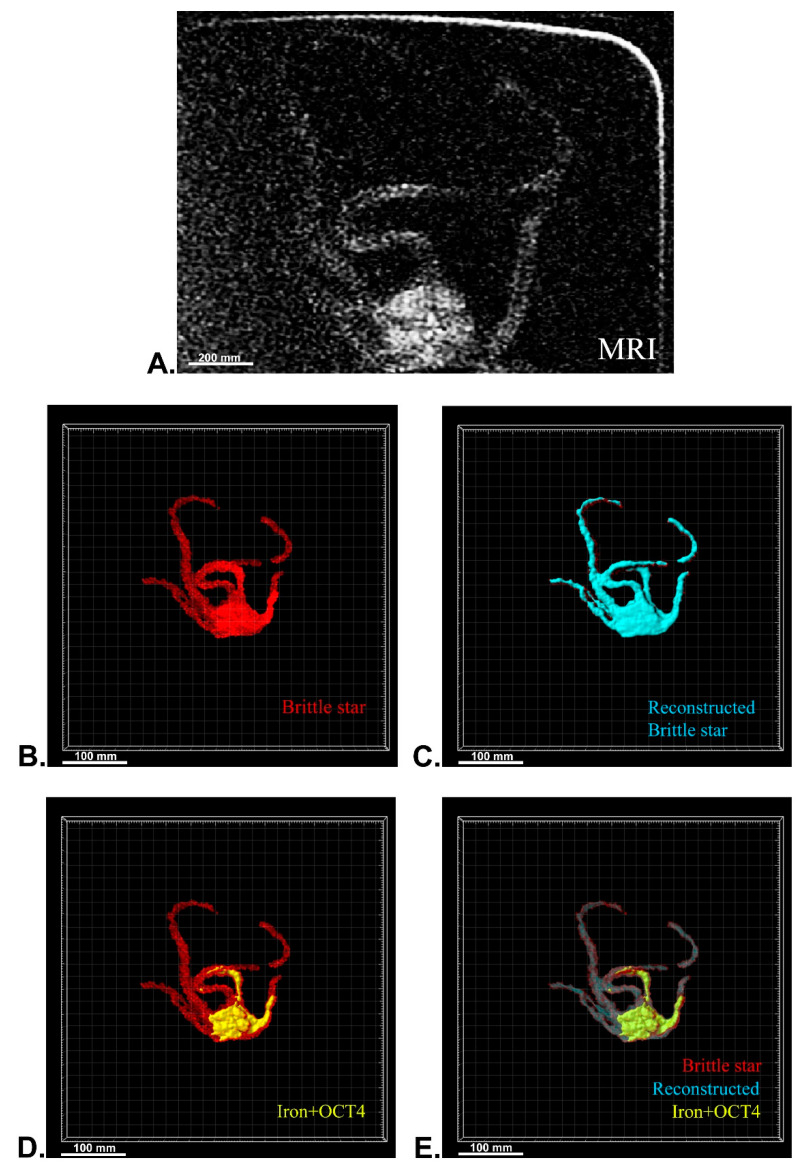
Three-dimensional (3D) reconstruction of brittle star tissue with iron plus anti-Oct4 antibody treatment using magnetic resonance imaging (MRI) serial images: (A) original grayscale image; (B) whole tissue imaging and signals of the brittle star tissue without reconstruction; (C) brittle star whole tissue 3D reconstruction in which the blue surface represents reconstructed brittle star tissue; (D) 3D reconstruction of the iron plus anti-Oct4 antibody signals (shown in yellow); (E) 3D reconstruction of the whole tissue of brittle star with its all signals. All scale bars in four images are 100 mm. All images were processed and generated by Imaris software (V 7.4.2, ImarisX64; Bitplane AG).
